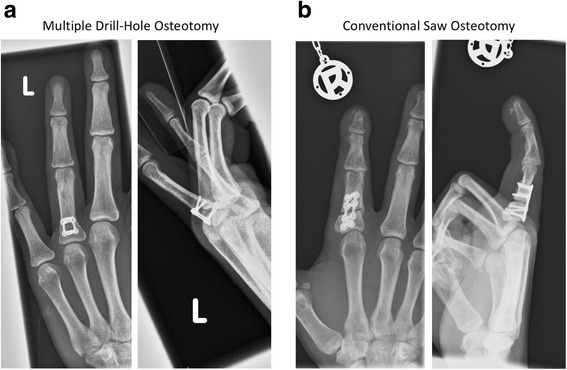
Comparison of Multiple Drill-Hole osteotomy (a) and conventional saw osteotomy (b). Pat. #7 treated with MDH osteotomy, at follow-up 3 months post-operative with good bony consolidation (a) compared to a patient treated with saw-osteotomy, radiographic study 3 months post-operative (b)
